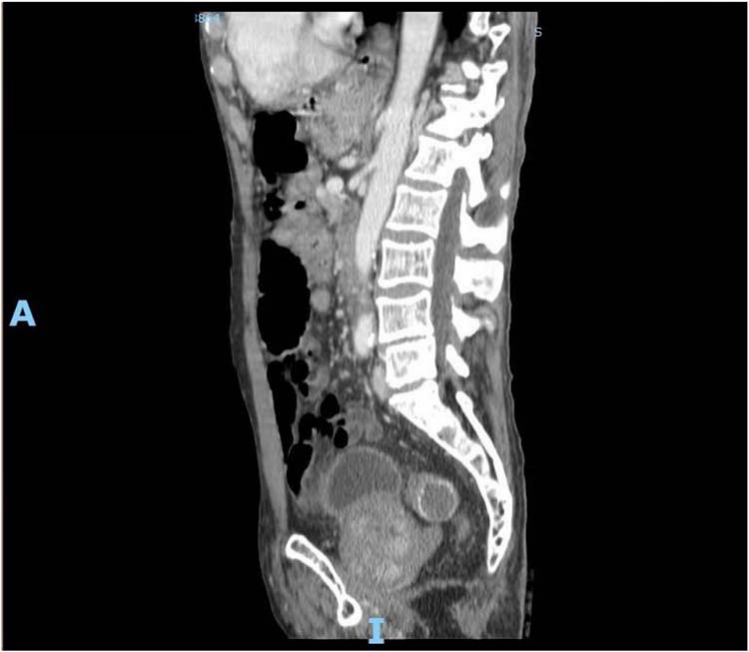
Enhanced CT scan of the abdomen showing a gallbladder stone impacted at the sigmoid colon, with a significantly enlarged prostate causing narrowing of the rectosigmoid junction (sagittal view).
